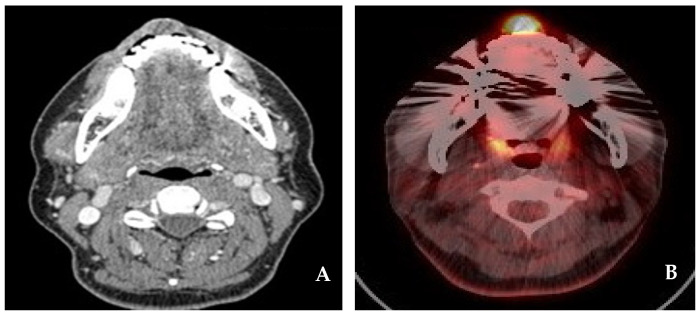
(A) CT neck(e). A well-defined hypodense mass with a size of 1.5 cm × 1.0 cm on the upper lip. (B) PET-CT. A focal metabolic lesion on the upper lip.
